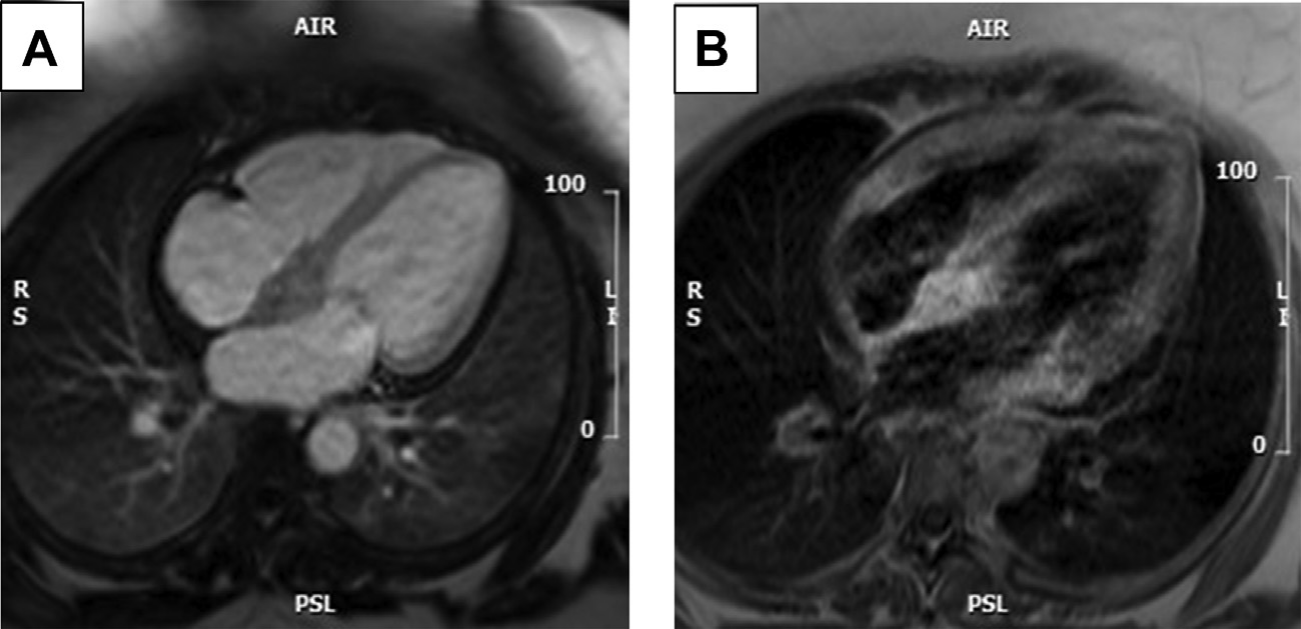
MRI With Gadolinium (A and B) showing low early gadolinium enhancement of the IAS and high signal late. Abbreviations as in Figure 2.
